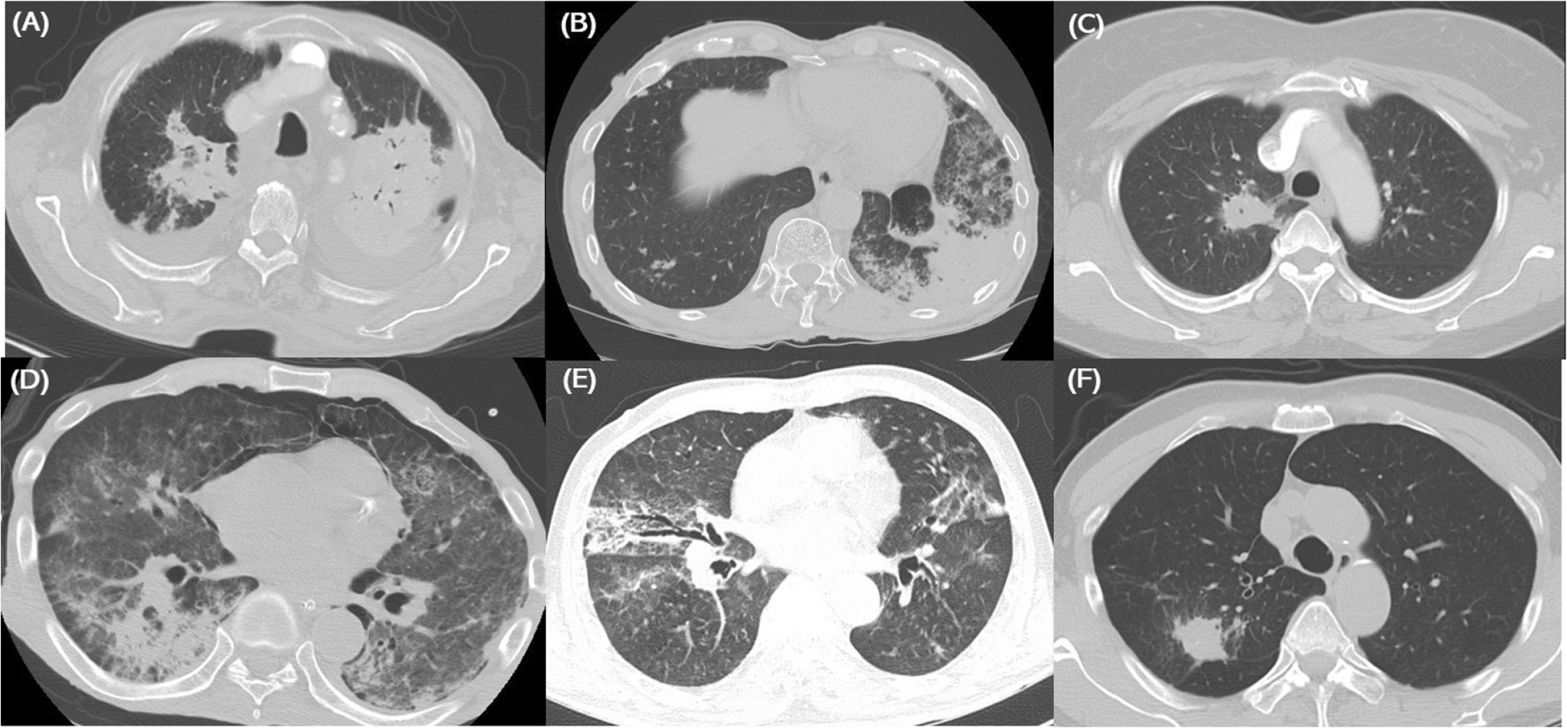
Chest computed tomography (CT) scan features of all six cases. a Case 1. Consolidation in both upper lobes and pleural effusion. Transbronchial lung biopsy (TBLB) was performed in the left upper lobe. b Case 2. Consolidation in the left lower lobe, which was subjected to TBLB. c Case 3. A thick-walled cavity is present in the right upper lobe, which was subjected to TBLB. d Case 4. Consolidation in the right lower and left lower lobes. TBLB was performed in the right lower lobe. e Case 5. Multiple nodules with patchy consolidation in both lungs. TBLB was performed in the right middle lobe. f Case 6. A solitary pulmonary nodule in the right upper lobe, which was subjected to TBLB
